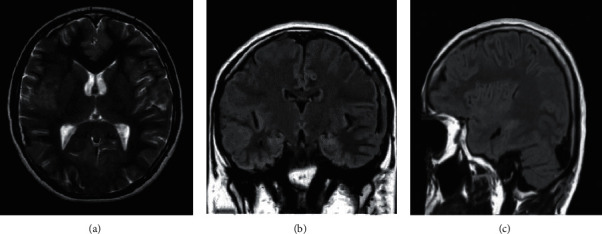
MRI images of healthy people.
